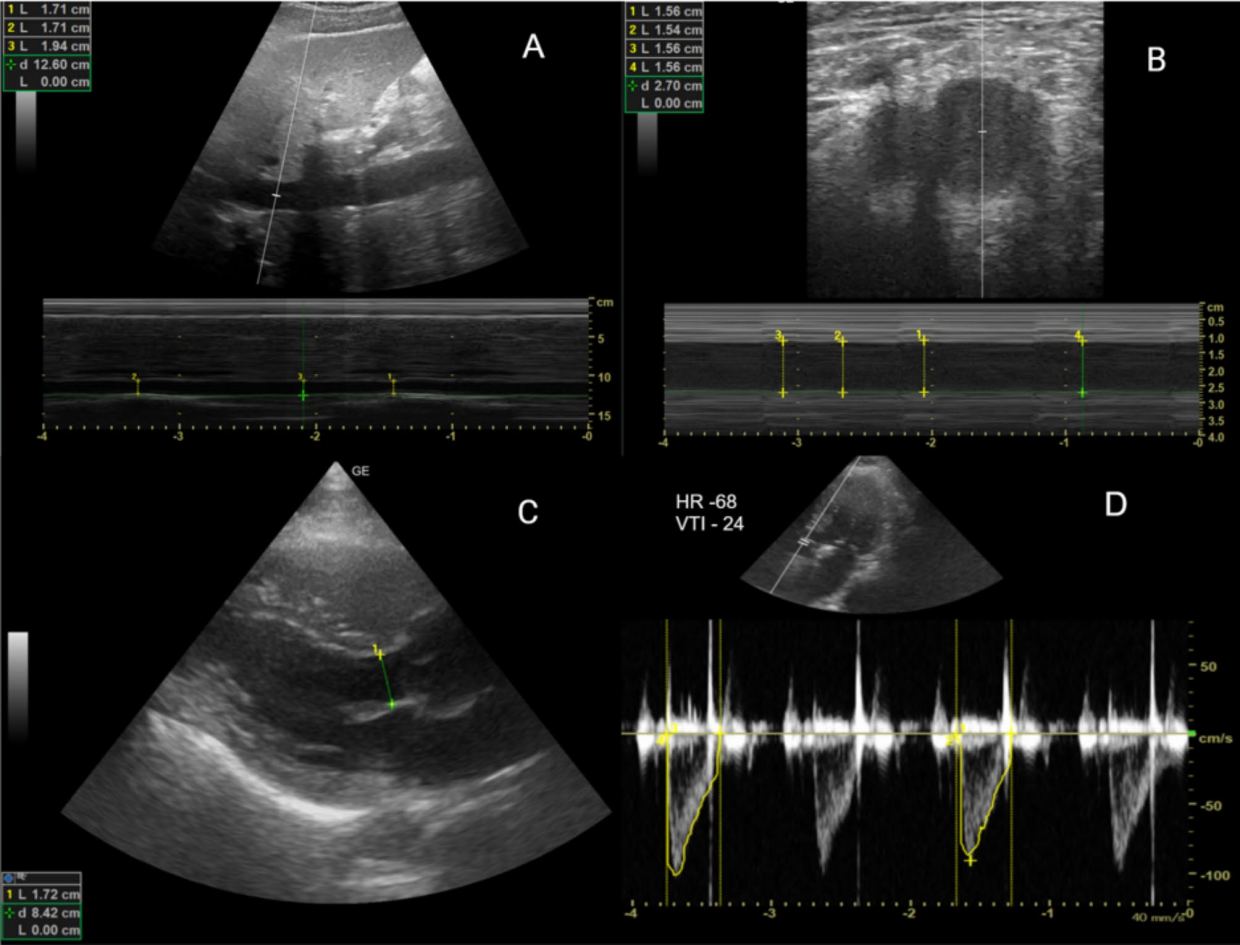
Ultrasound images of IVC, femoral vein & LVOT-VTI Image A -Ultrasound image of IVC using M mode. L1, L2, L3– 3 values of IVC diameters; d – depth at which IVC is measured Image B - Femoral artery(left), Femoral vein(right) L1, L2, L3, L4 – Diameters of femoral vein on inspiration and expiration; d – depth at which femoral vein is measured Image C - Ultrasound image of parasternal long axis view of LVOT; L – diameter of LVOT; d – depth at which LVOT is measured Image D - Ultrasound image of LVOT with VTI measurement in apical 5 chamber view VTI Velocity time integral of 24; HR – Heart rate of 68 bpm
